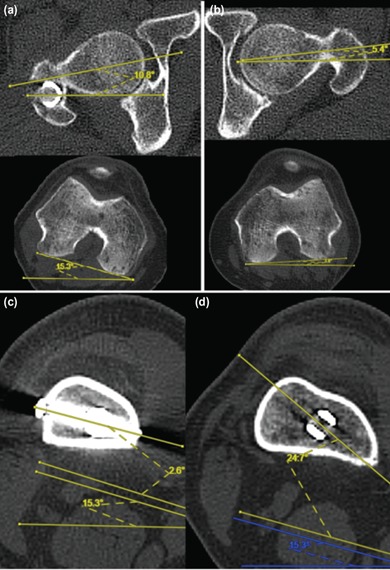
CT scan demonstrated right femur had external malrotation, and the nail placement was excessively internal rotated. (a) Right femur had 26.1° anteversion (10.8 + 15.3), (b) uninjured left femur had 1.6° retroversion (-5.4 + 3.8), (c) axial cut of right distal femur at level of static screw hole showed that the static screw was parallel to transcondylar axis, (d) axial cut of right distal femur at level of dynamic screw holes showed that the dynamic screw hole was 25° internal rotated in relation to transcondylar axis.
